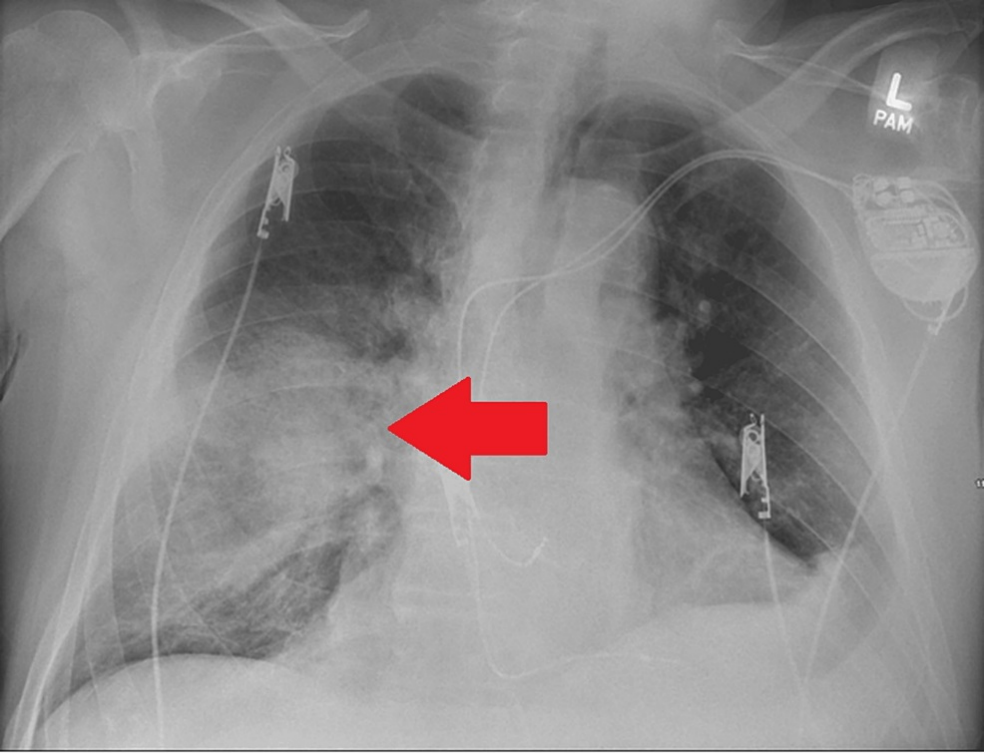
Posteroanterior chest radiograph showing perihilar consolidative opacity in the right lower lobe.
